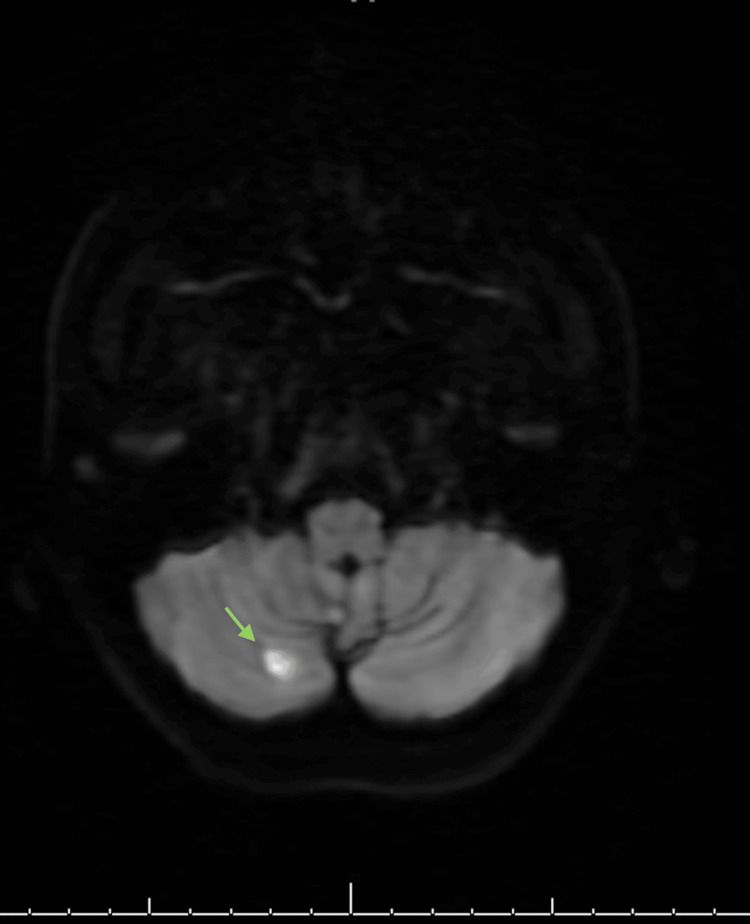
MRI of the head with contrast was done, indicating an acute/subacute ischemic stroke in the right cerebellar hemisphere.
